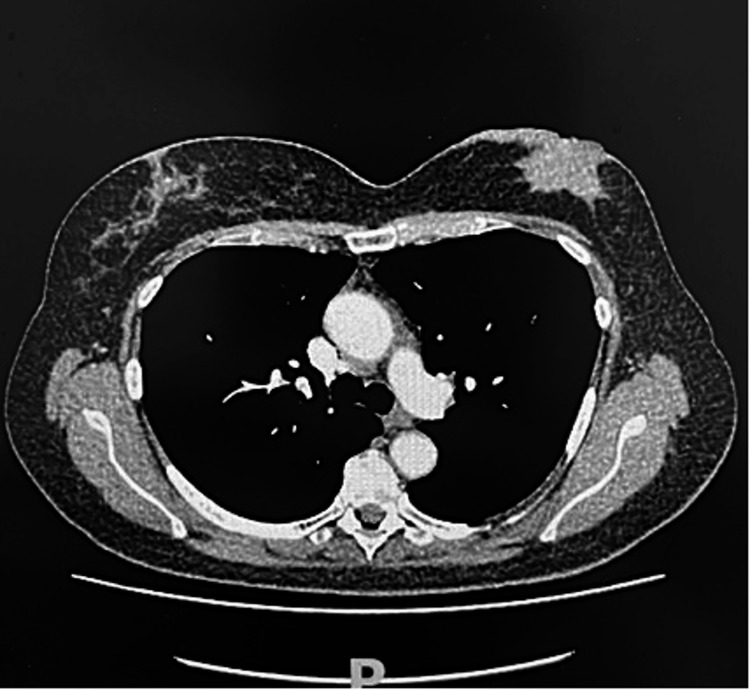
CT images demonstrating fungating locally advanced left-sided breast cancer with skin invasion.
